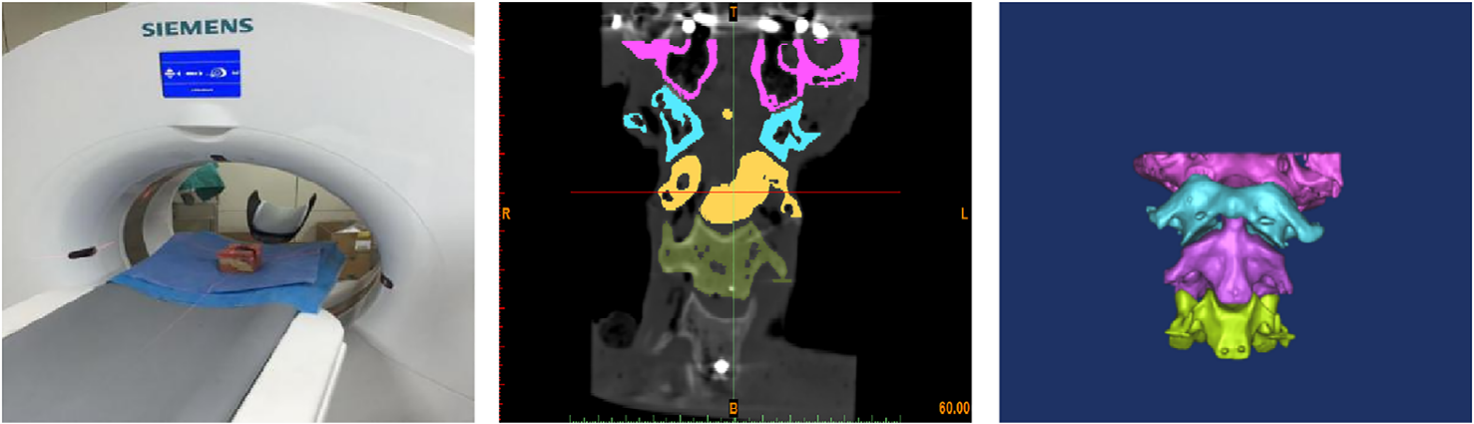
The CT machine was used to scan the upper cervical spine. Specifically, 219 tomograms were saved in the dicom format. In the segmentation module, the bone structure was selected by threshold selection in order to draw, erase, calculate three dimensions, smooth surfaces to perform other editing processes, and to obtain the corresponding position of the mark.
